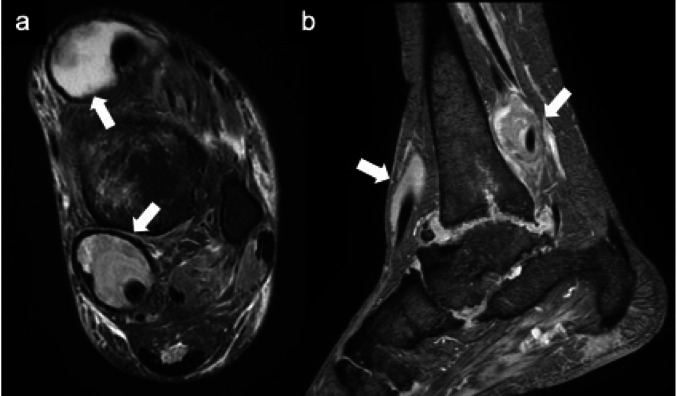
Rheumatoid arthritis Axial fat-suppressed T2-weighted MR image (a) and sagittal T2*-weighted MR image (b) show distention of the tendon sheaths (arrows). The high signal intensity surrounding the tendons indicates fluid accumulation within the tendon sheaths, suggesting tenosynovitis
